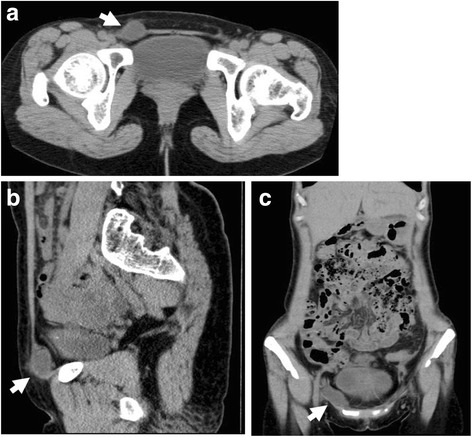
Computed tomography scans showing a round cystic lesion (*arrow*) within the right inguinal canal. a Transverse image. b Sagittal image. c Frontal image
